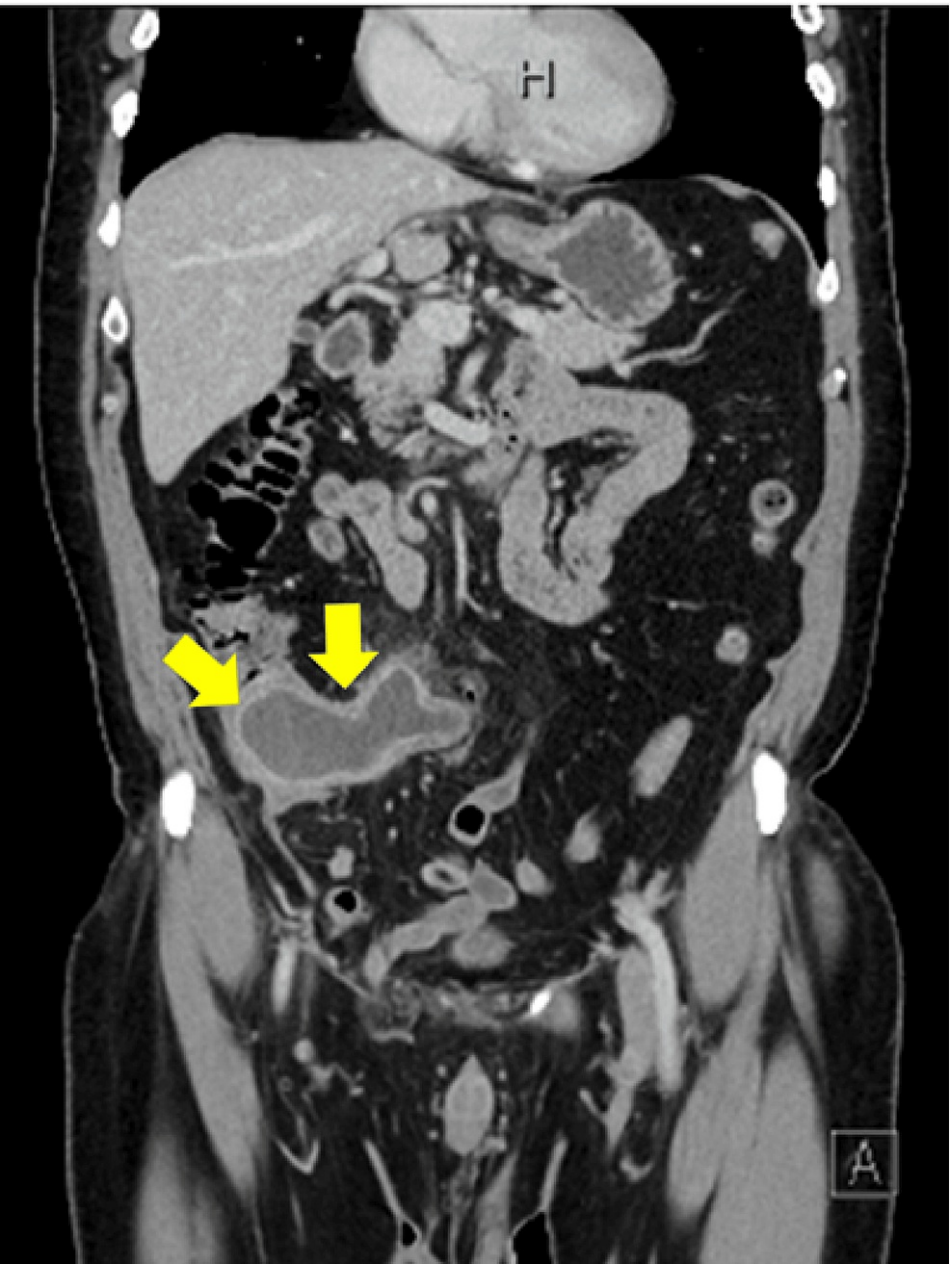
Contrast-enhanced computed tomography at the present time (coronal image).
